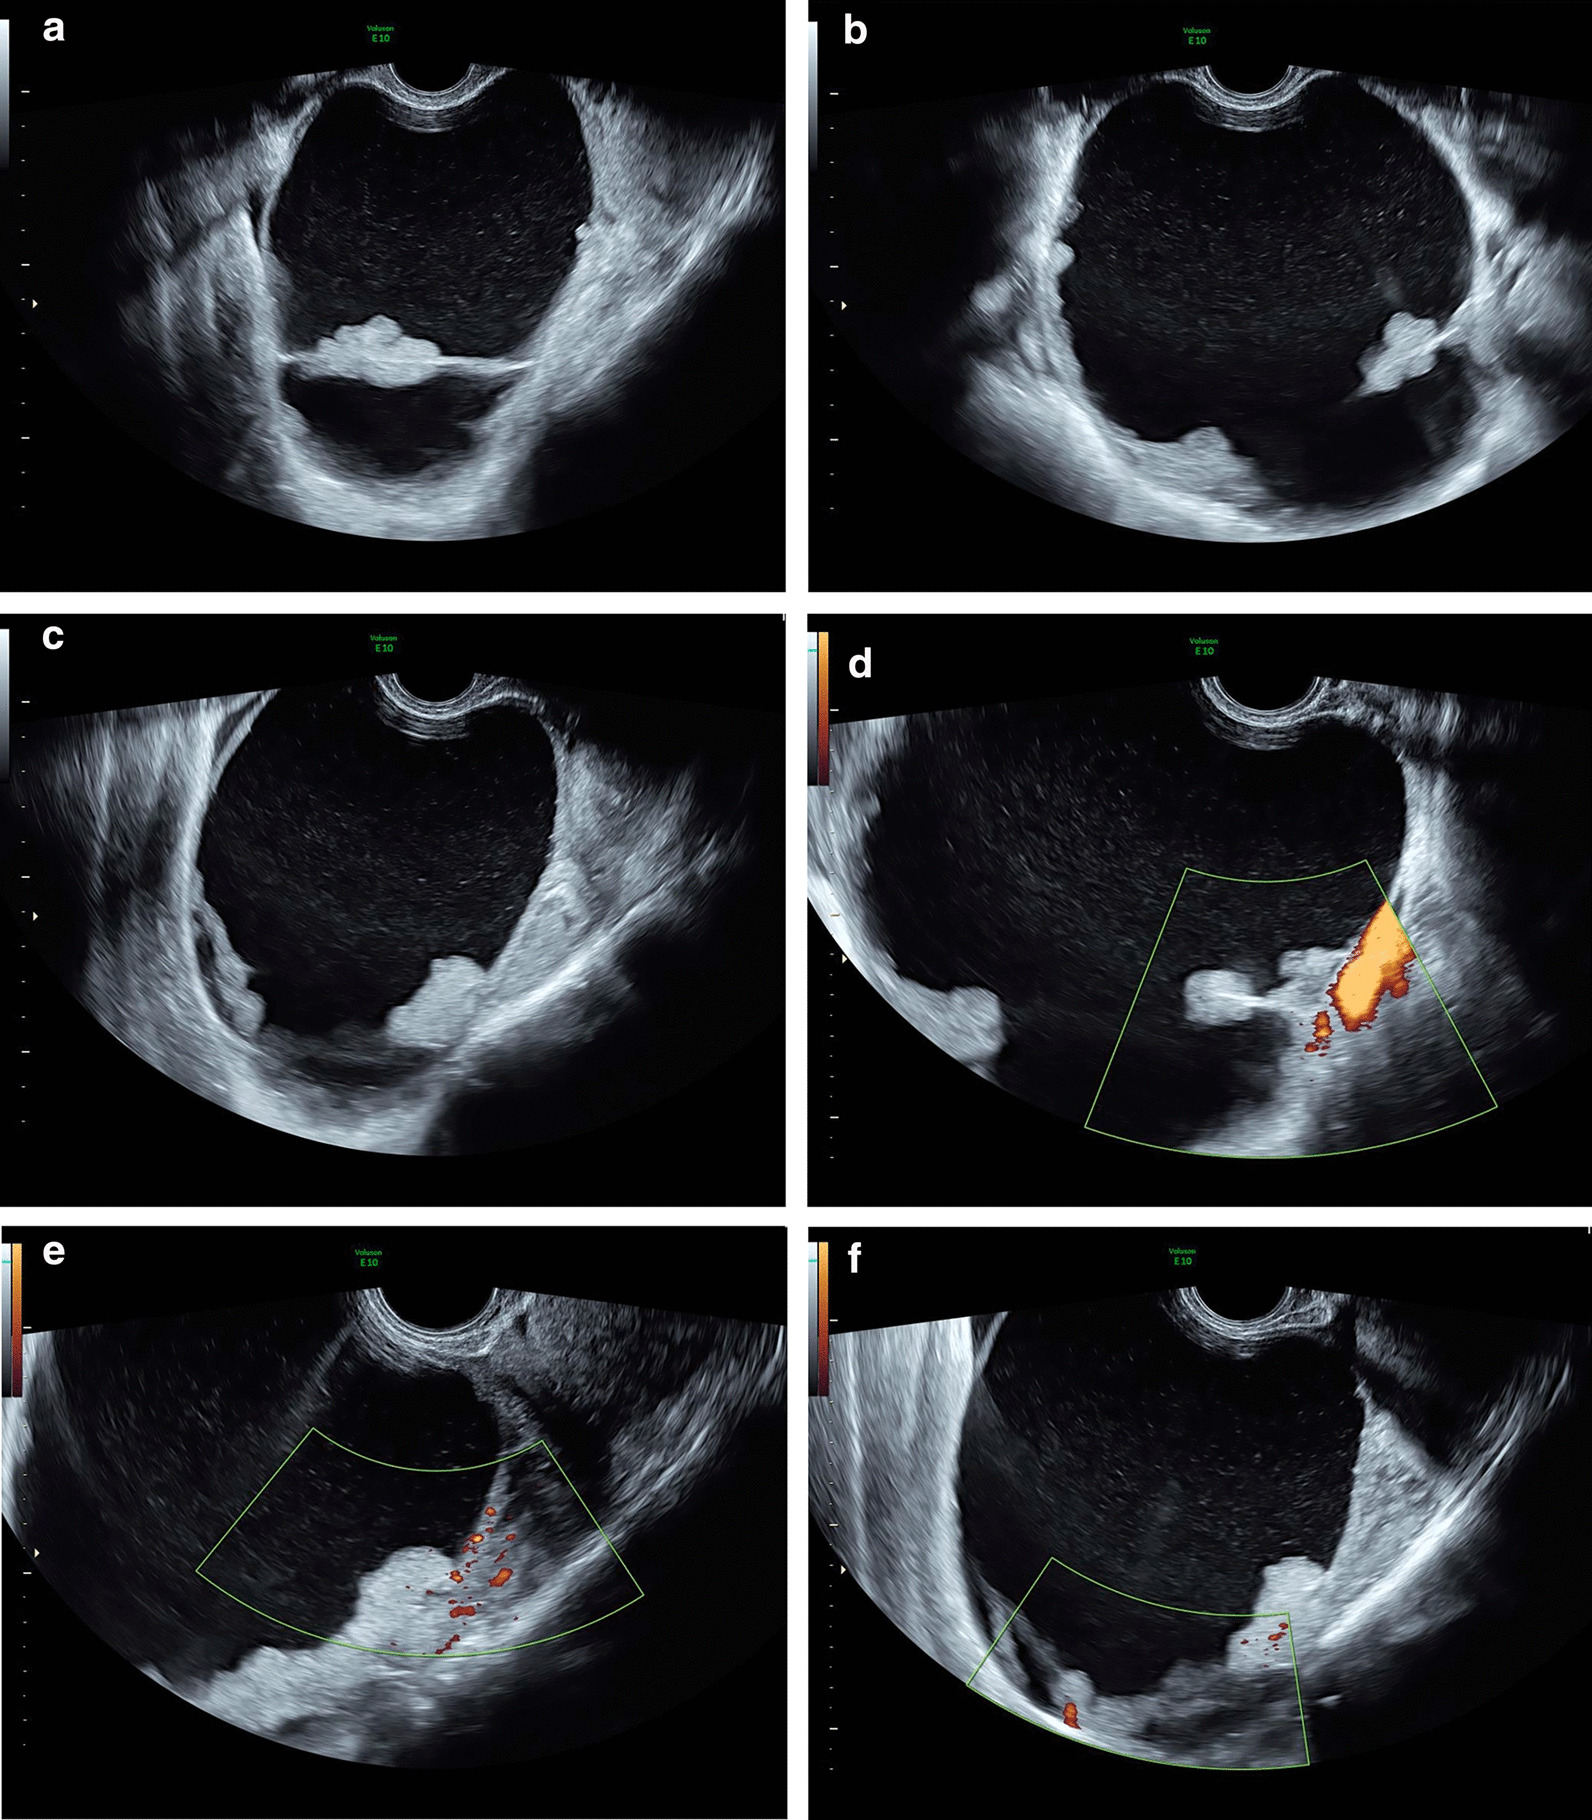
Grayscale (a–c) and color Doppler (d–f) transvaginal sonography: right ovary multilocular-solid mass, with papillary excrescences and low vascularization
